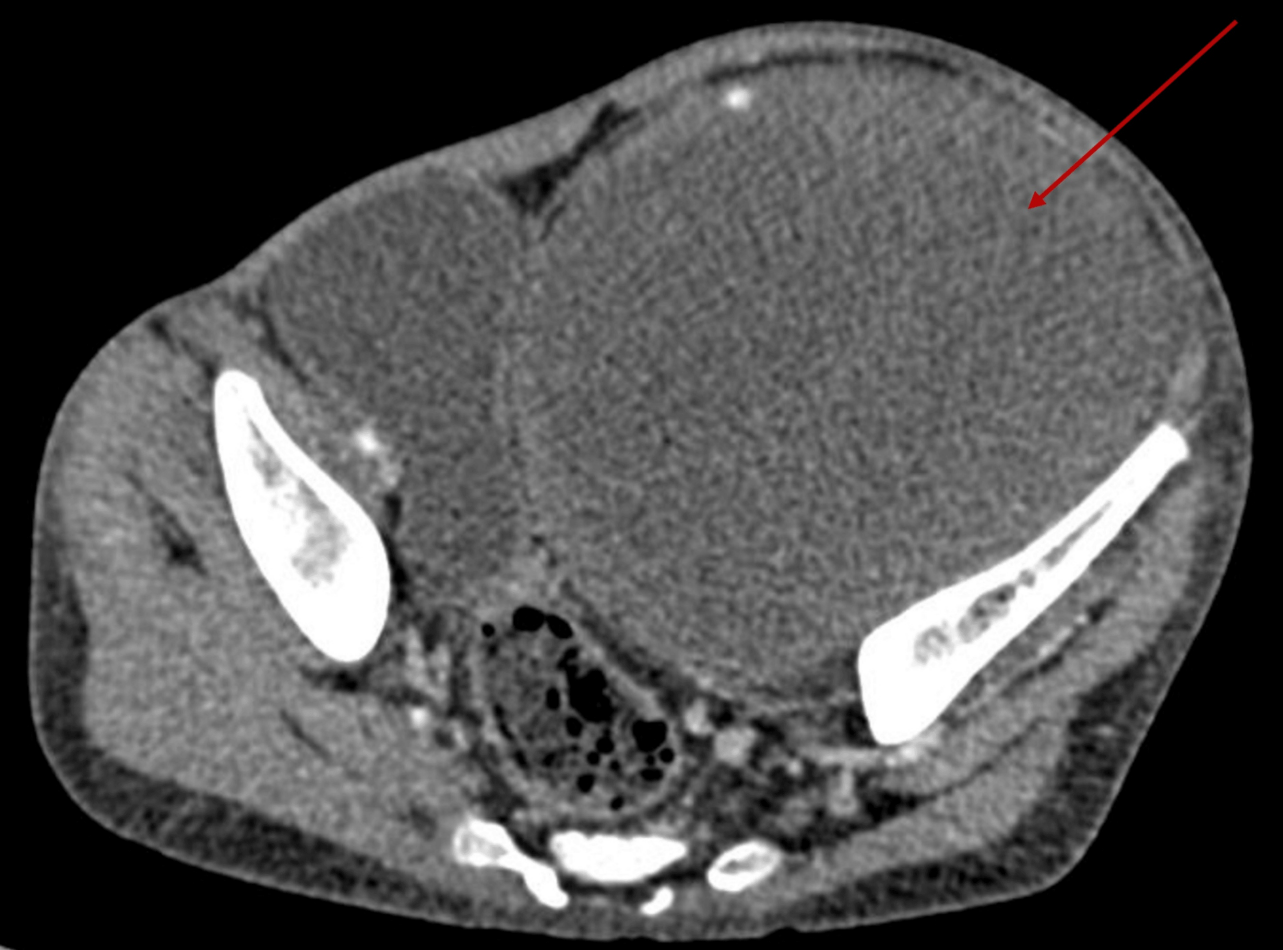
Axial abdominopelvic CT demonstrating a large pelvic soft tissue mass. Axial abdominopelvic CT image showing a large heterogeneous pelvic soft tissue mass (red arrow), occupying most of the pelvis and causing mass effect on adjacent structures.
